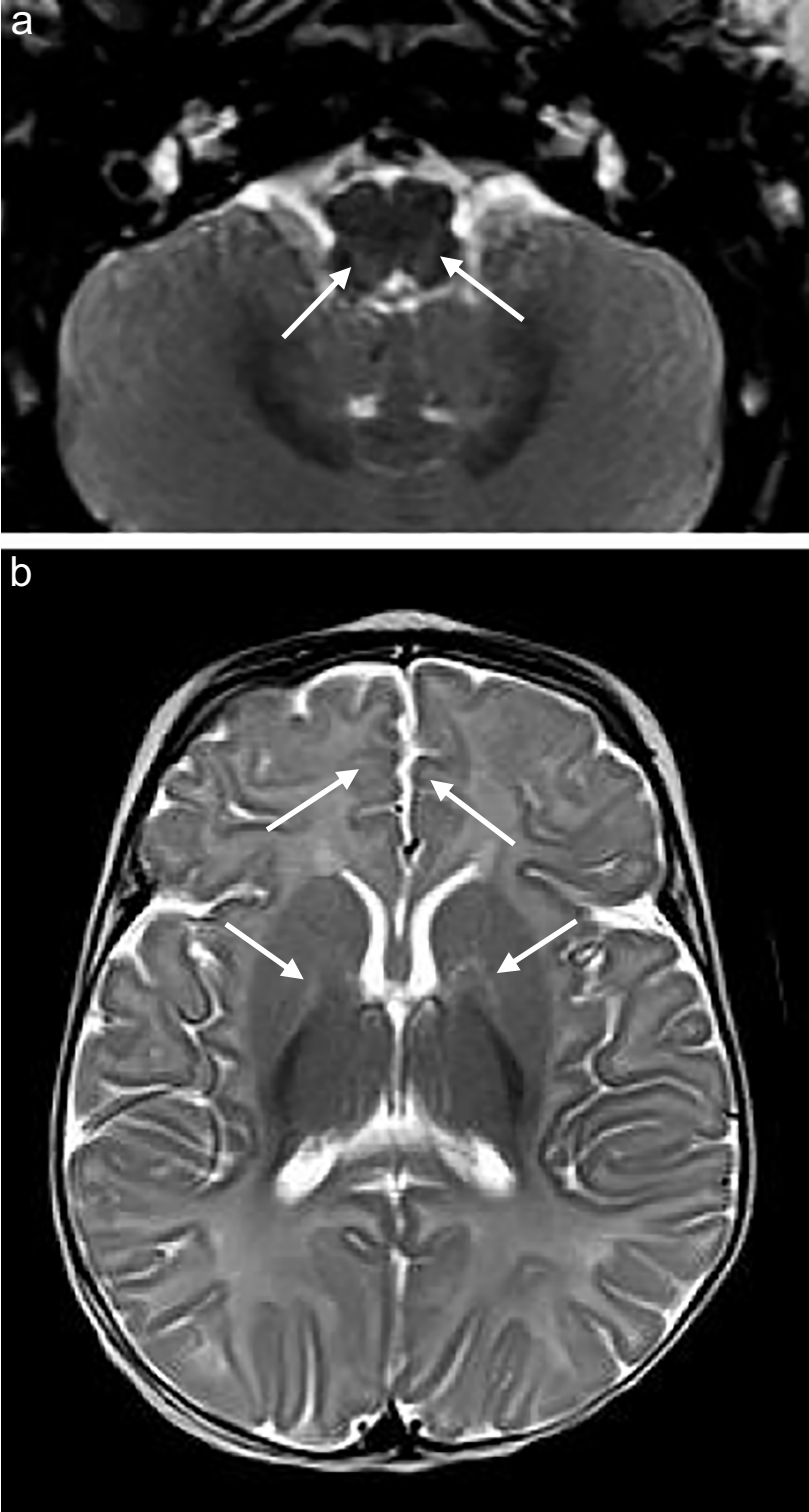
A 4-month-old female with genetically confirmed *MPV17*-related mitochondrial depletion syndrome. a Axial T2-weighted image shows hyperintense signal in the reticular formation of the medulla (*arrows*). b Axial T2-weighted image shows hyperintense signal in the posteromedial globus pallidi (*arrows*)
